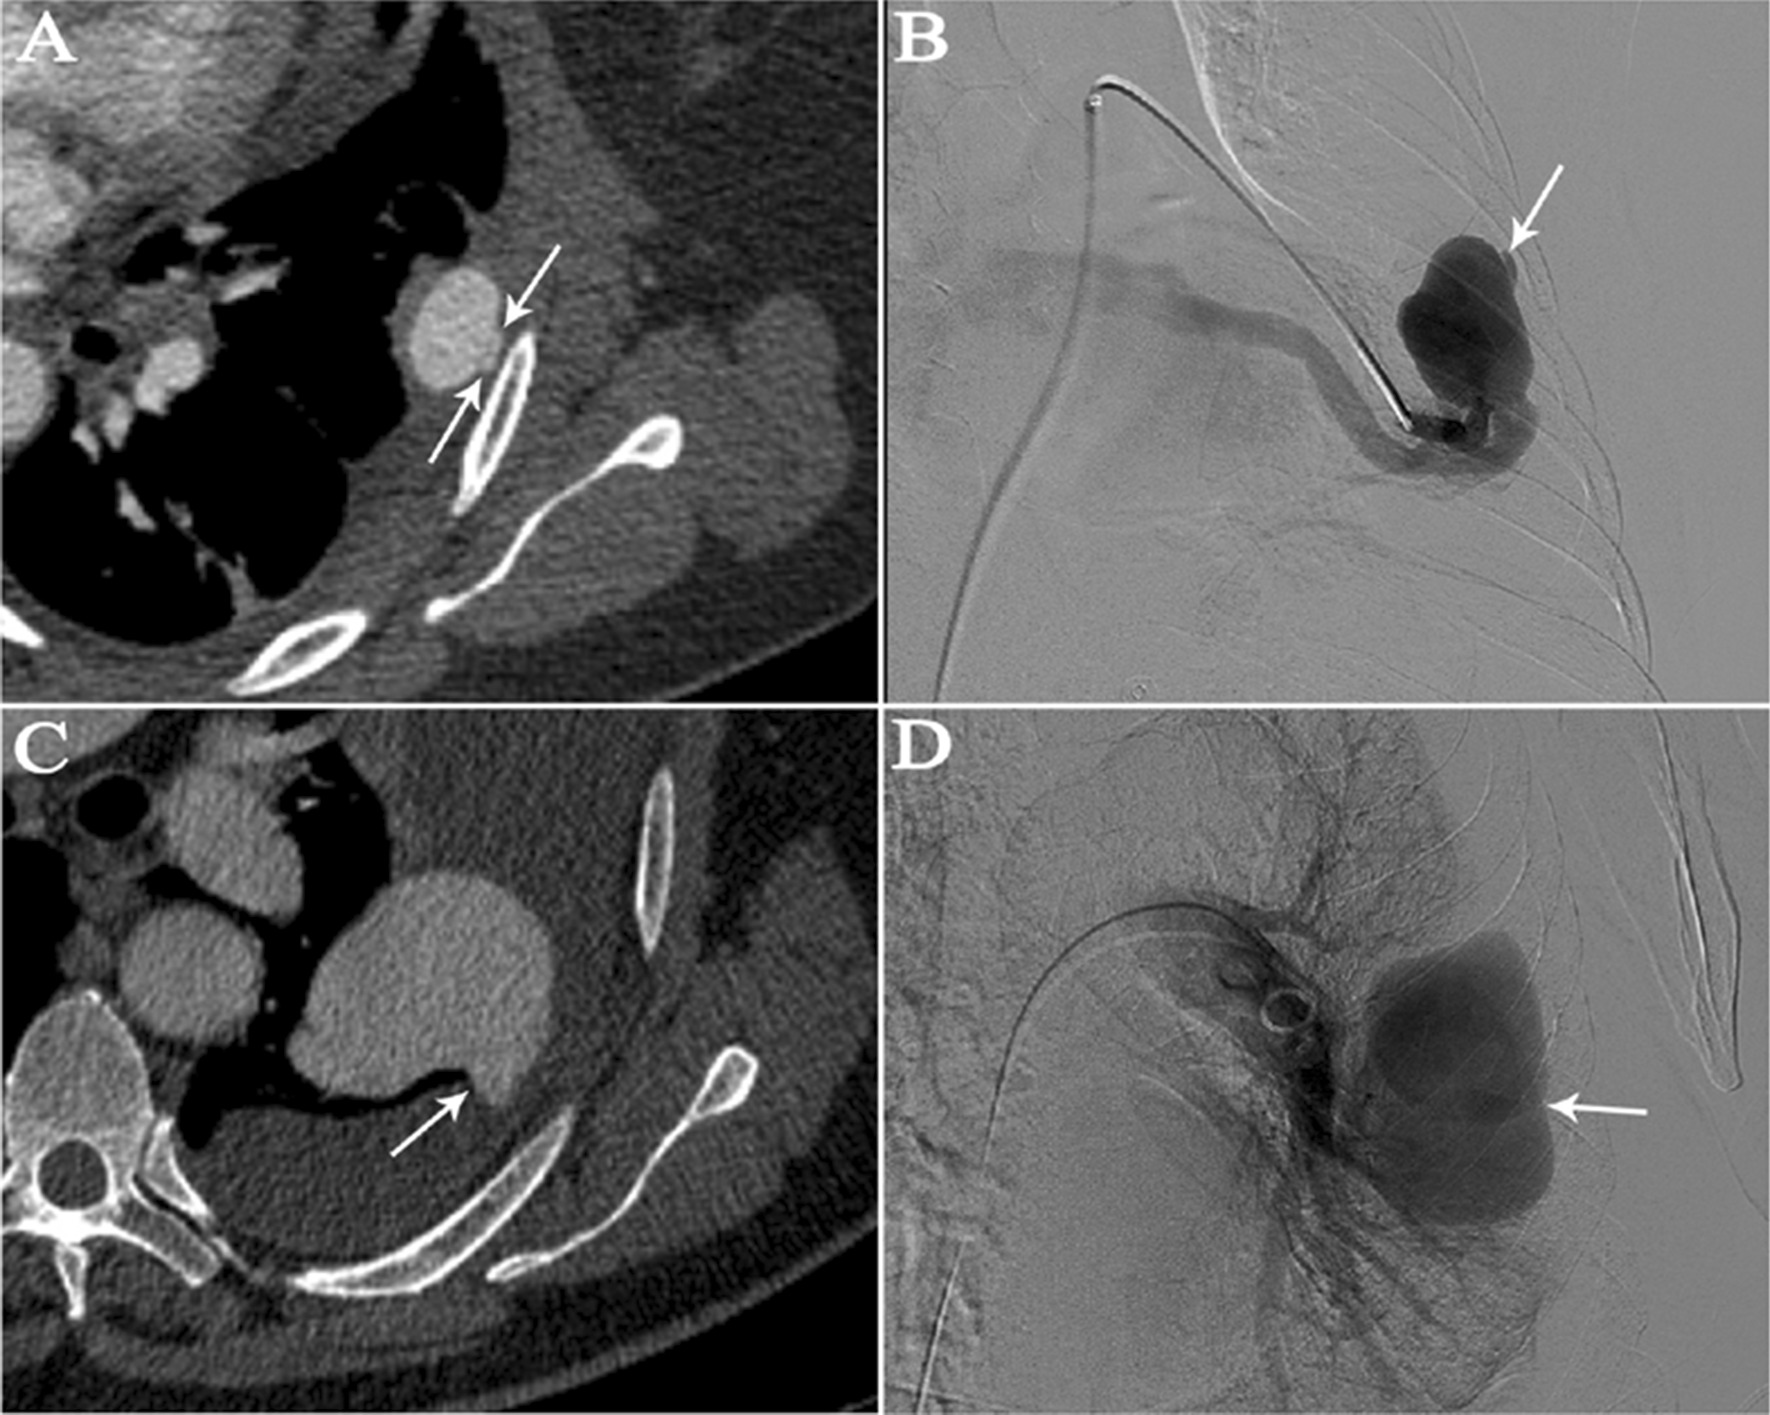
A, B a 47-year-old woman with haemothorax; C, D a 72-year-old man with haemothorax. A, C “Anomalous bulge” sign on CTA: vessel wall of ruptured PAVM and (supposedly) pulled towards the pleura by the negative intrathoracic pressure (arrows). B, D the overlap of “anomalous bulge” and the adjacent PAVM’s sac gives rise to the “double shadow sign” on the 2D-angiography (arrows)
